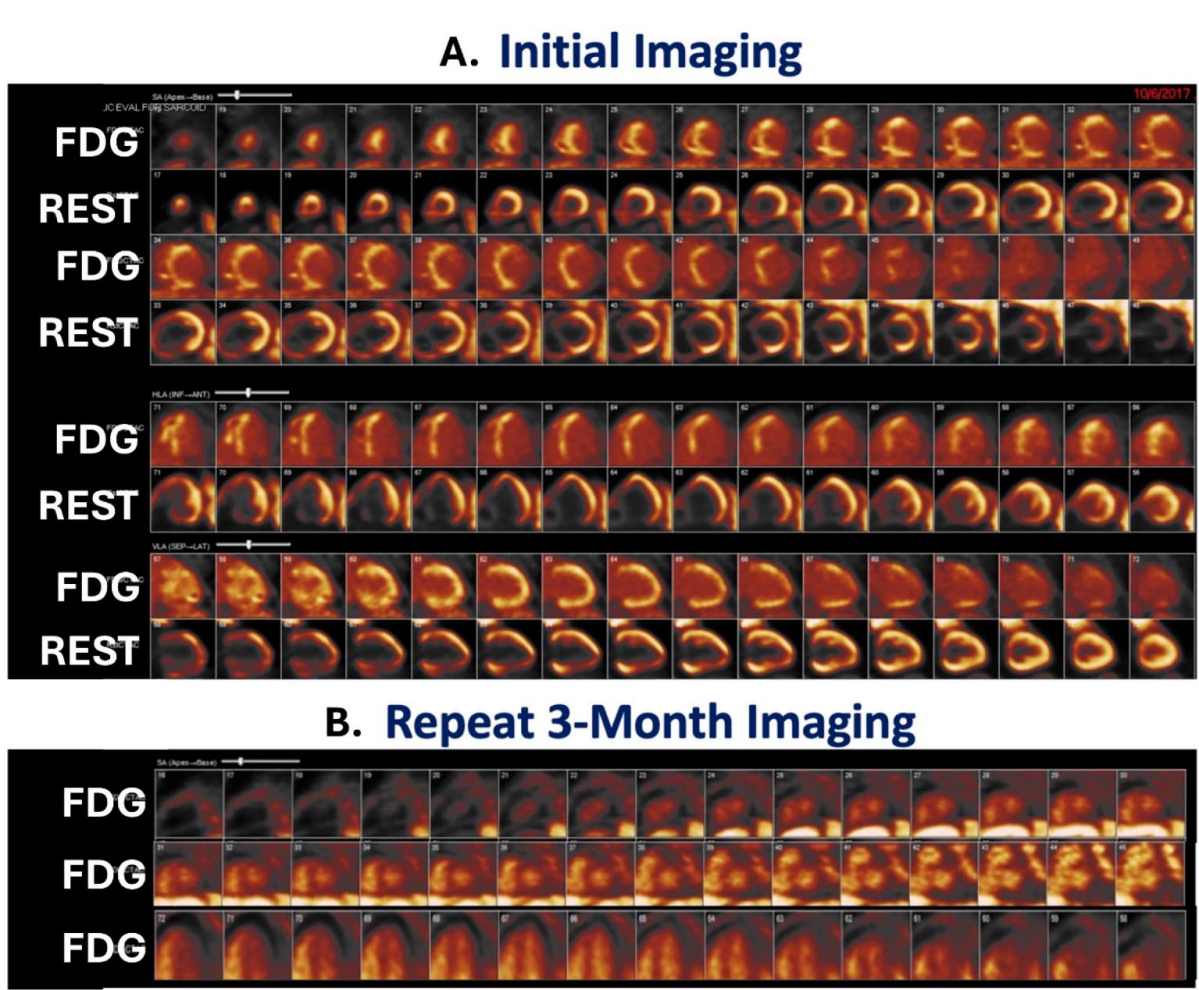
Serial ^18^F-FDG PET/CT imaging to diagnose cardiac sarcoidosis and assess response to therapy. A 67-year-old female with a history of pulmonary sarcoidosis presents with an episode of paroxysmal ventricular tachycardia after three weeks of dyspnea on exertion and orthopnea. She underwent positron emission tomography/computed tomography with ^18^F-fluorodeoxyglucose (FDG) to assess inflammation and ^13^N-ammonia to evaluate perfusion (A). Imaging revealed a high concern for active inflammation from cardiac sarcoidosis with prominent heterogeneous ^18^F-FDG uptake in the apical to basal anteroseptum, inferoseptum, and inferior walls and associated perfusion defect in the same territory. She was started on prednisone and transitioned to mycophenolate mofetil and heart failure goal-directed medical therapy. She had resolution of her symptoms. Repeat imaging at 3 months showed complete resolution of her ^18^F-FDG uptake consistent with disease remission (B). She was able to taper off therapy successfully
